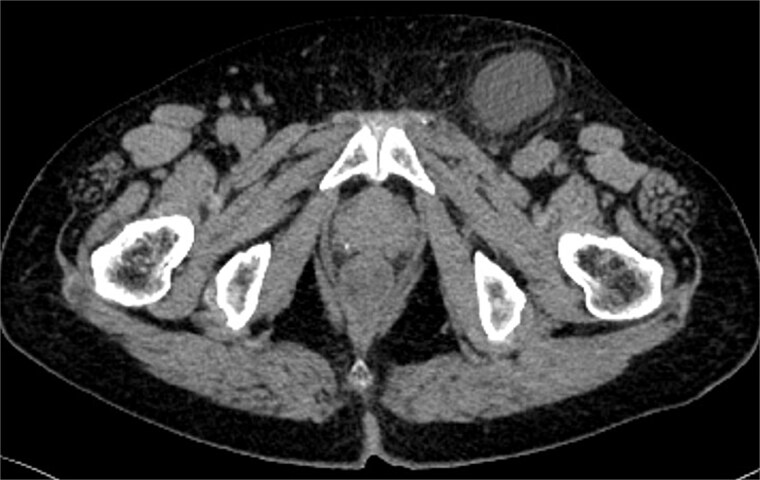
CT findings in Case 2. CT image showing a 4-cm left inguinal hydrocele.
